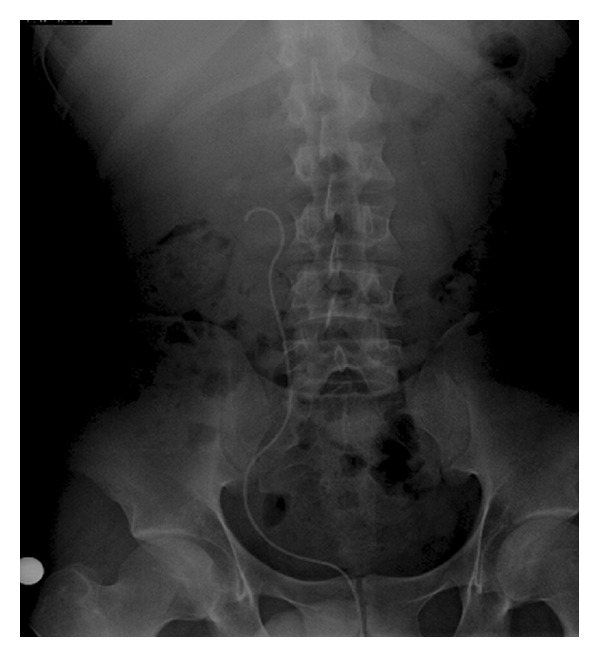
Kidney, ureter, and bladder (KUB) X-ray study showing migration of the double J stent into the urethra after extracorporeal shock wave lithotripsy (ESWL).
